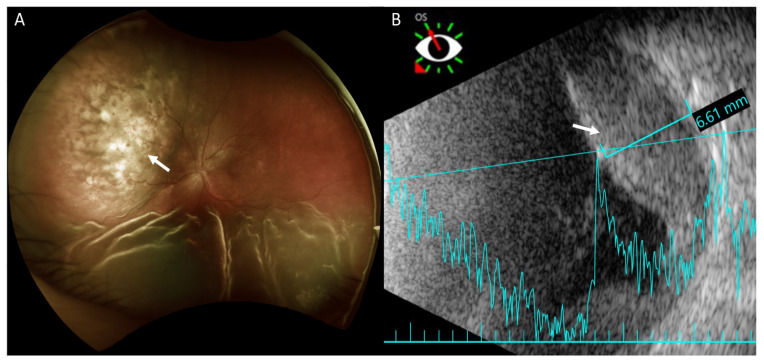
Fundus photography and B-scan ultrasonography of the left eye. (A) Fundus photography shows exudative retinal detachment in the inferior retina and an amelanotic choroidal mass in the superior quadrant (arrow) in marked relief. Exudative retinal detachment is seen mainly on the sides of the choroidal mass. (B) Ultrasonography shows the achromic mass in the superior region as a hyperechoic lesion in the subretinal region (arrow) with a thickness of approximately 6.61 mm.
